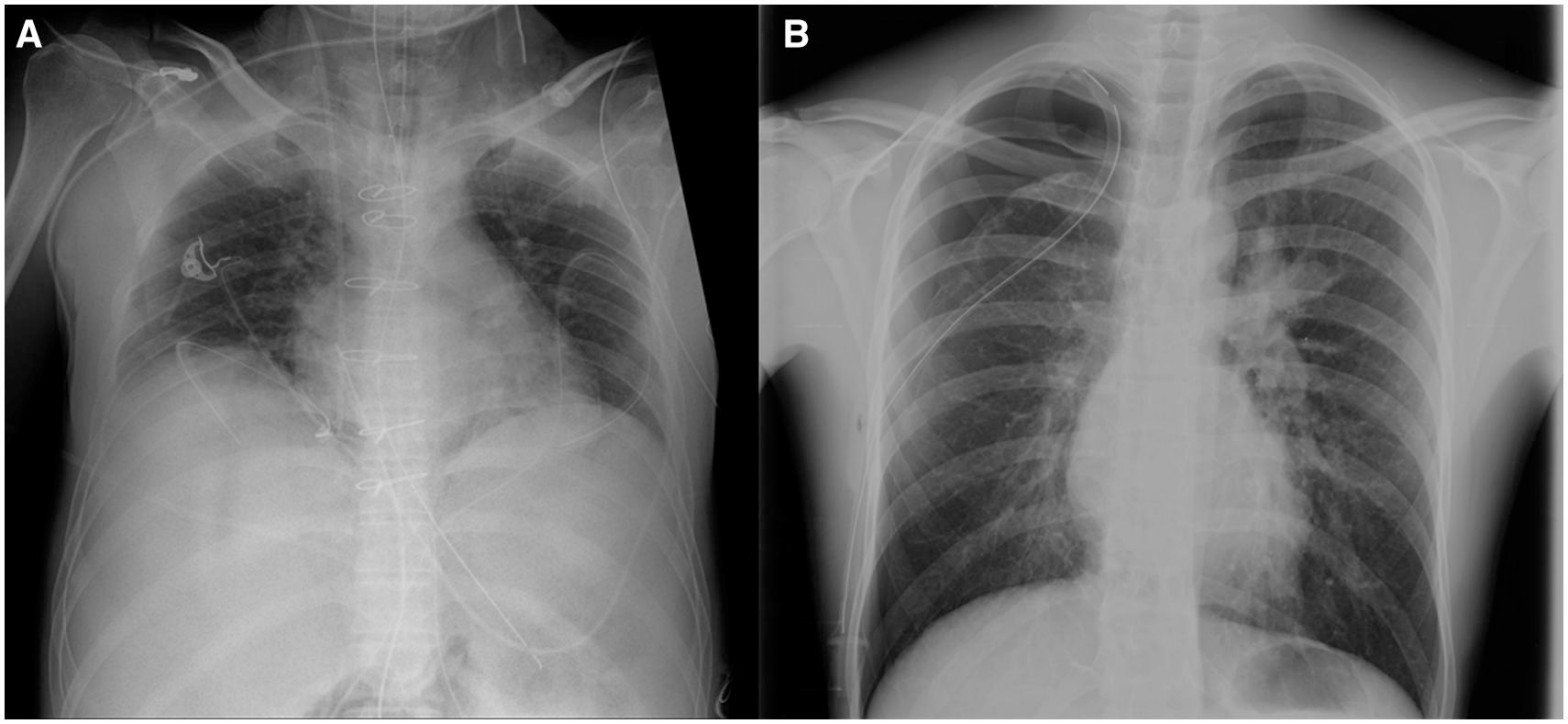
Chest X-rays of ICU patients. (A) Chest X-ray acquired using improper X-ray beam collimation, as the upper abdomen is included, with a consequent increase in dose–area product and effective dose. Multiple devices are present, including a central venous catheter, tracheal tube, nasogastric tube, and chest tubes. (B) X-ray beam collimation was appropriately limited in this patient, as only the chest region is included. A pneumothorax is visible on the right apex, drained by a chest tube.
